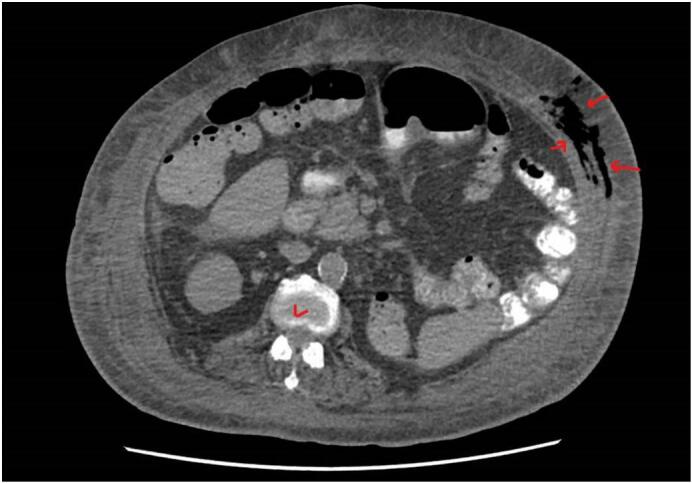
Axial CT abdomen with air in the abdominal wall (arrows); V vertebral column.
